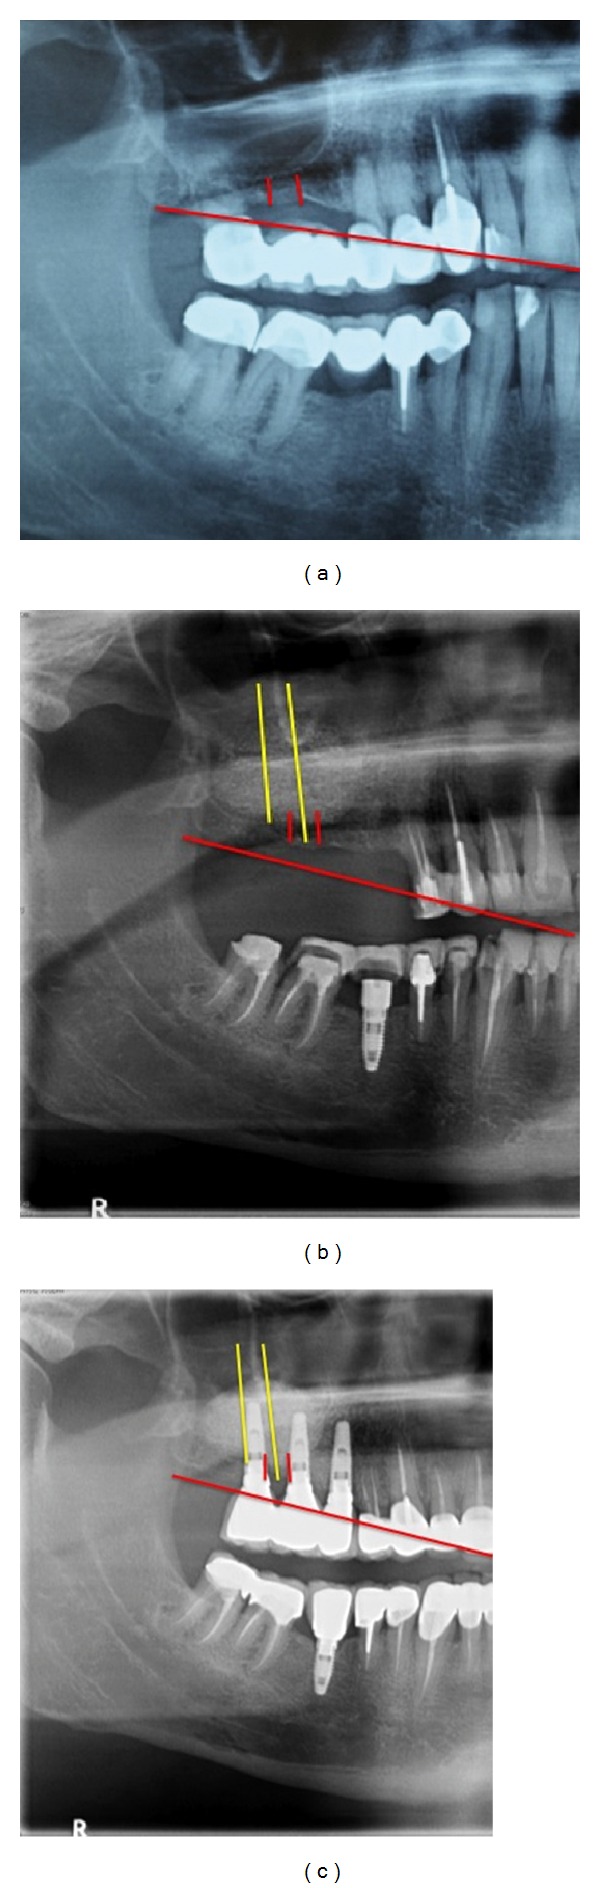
Panoramic X-ray with fixed measuring points at base line (a), after grafting procedure using Cerabone (b), and four years of implant placement (c).
